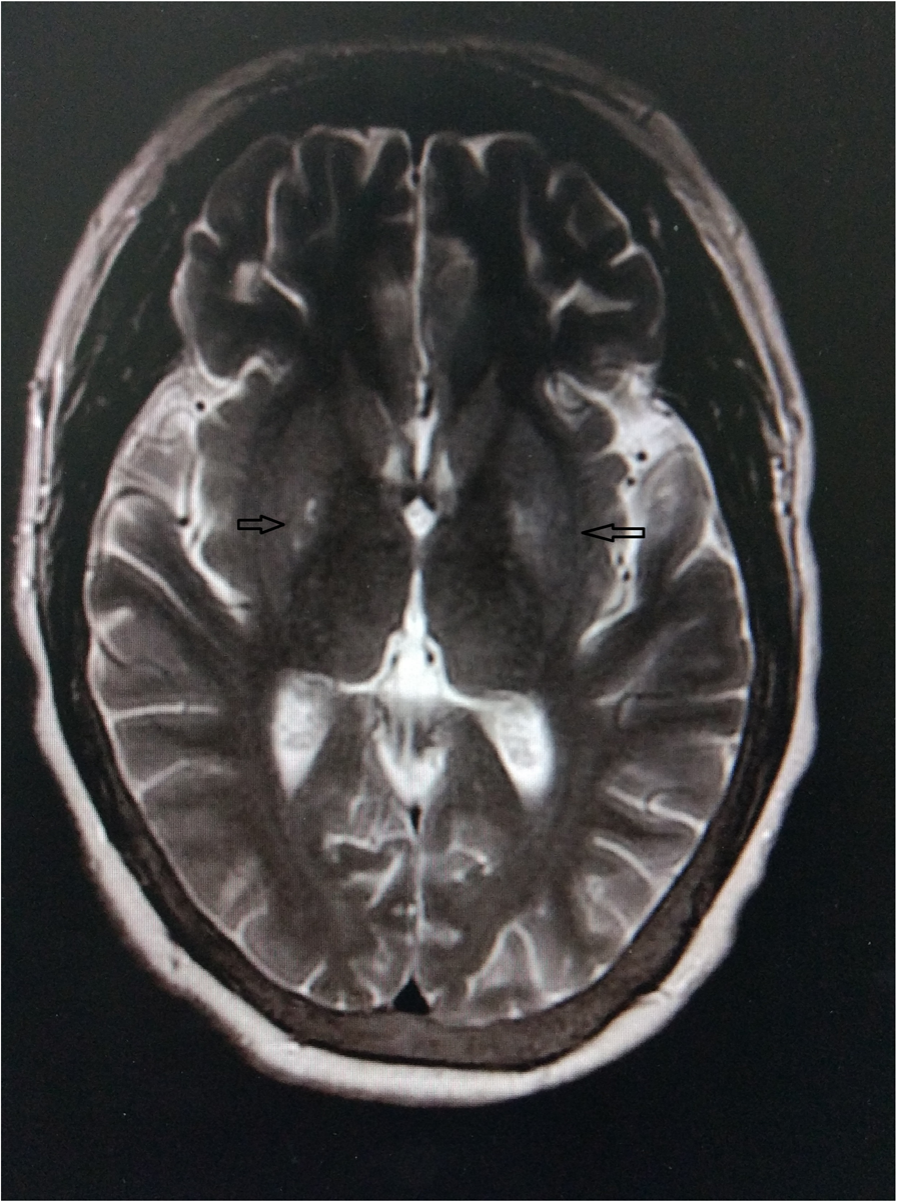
Follow-up MRI scan obtained after 14 days. T2WI revealed high signal in the bilateral basal ganglia (arrows), which were significantly weaker compared to the initial MRI signal
